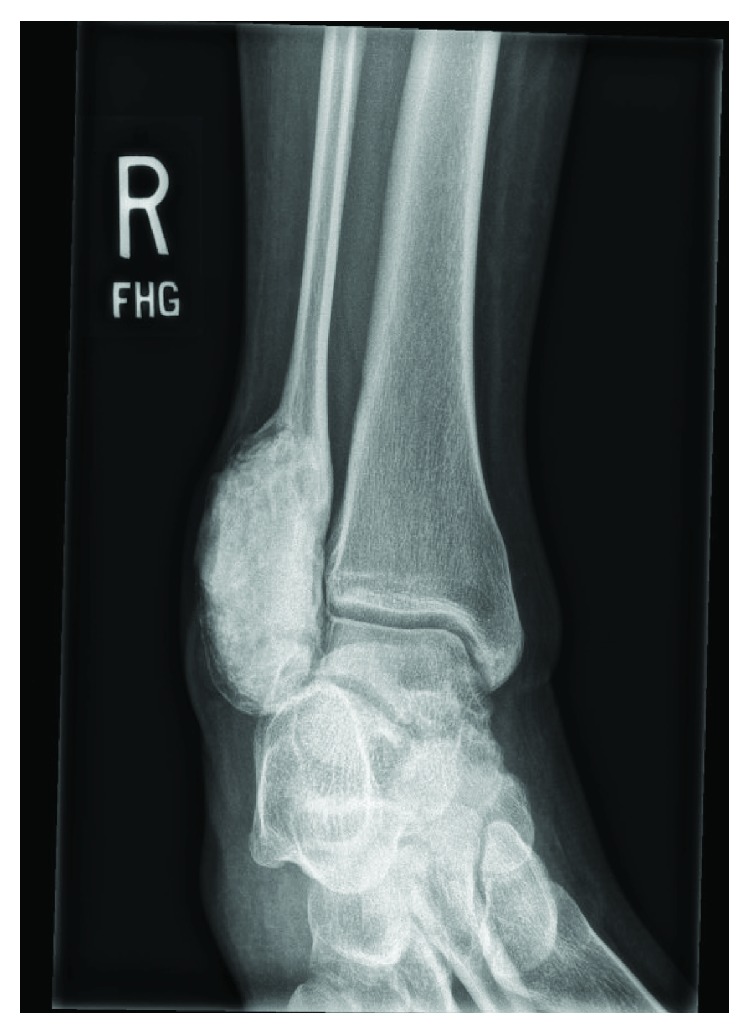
Mortise radiographs taken at 10 months following denosumab treatment and surgery demonstrating no local recurrence.
